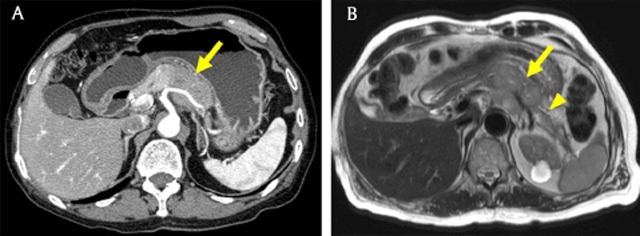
A 67-year-old man with prostate cancer. (A) Axial pancreatic phase CT image shows a low attenuating mass (arrow) encasing the splenic artery in the pancreatic body. (B) Axial T2-weighted image shows hyperintense mass (arrow) and normal pancreatic duct (arrowhead) without upstream dilatation.
